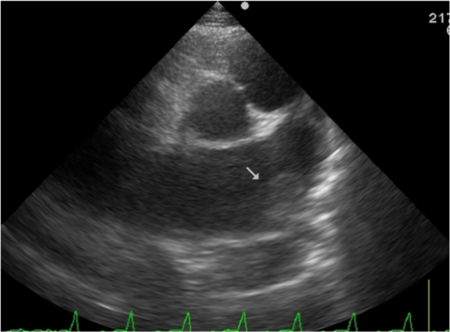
Echocardiograph demonstrating a thrombus-like echo in the left atrium (*white arrow*)
